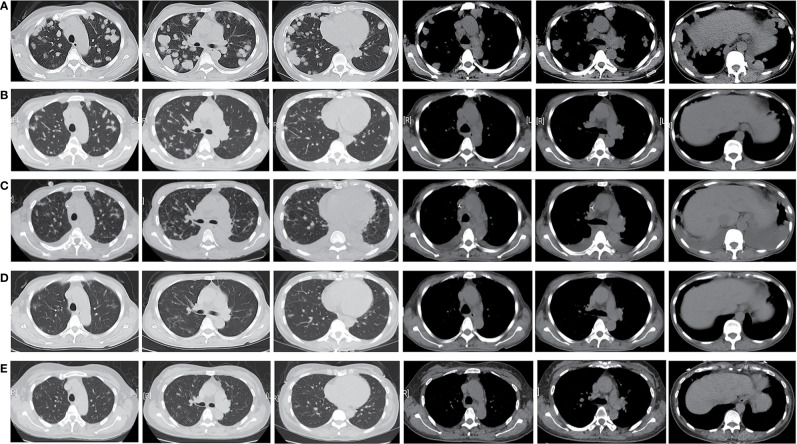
Timeline of the patient’s chest CT scan showed the obvious remission of the patient’ s diffused lesions in the bilateral lung and enlarged lymph nodes after crizotinib treatment: (A) 7 July, 2020 (baseline), (B) 24 August, 2020, (C) 22 September, 2020, (D) 14 December, 2020, (E) 25 April, 2021.
